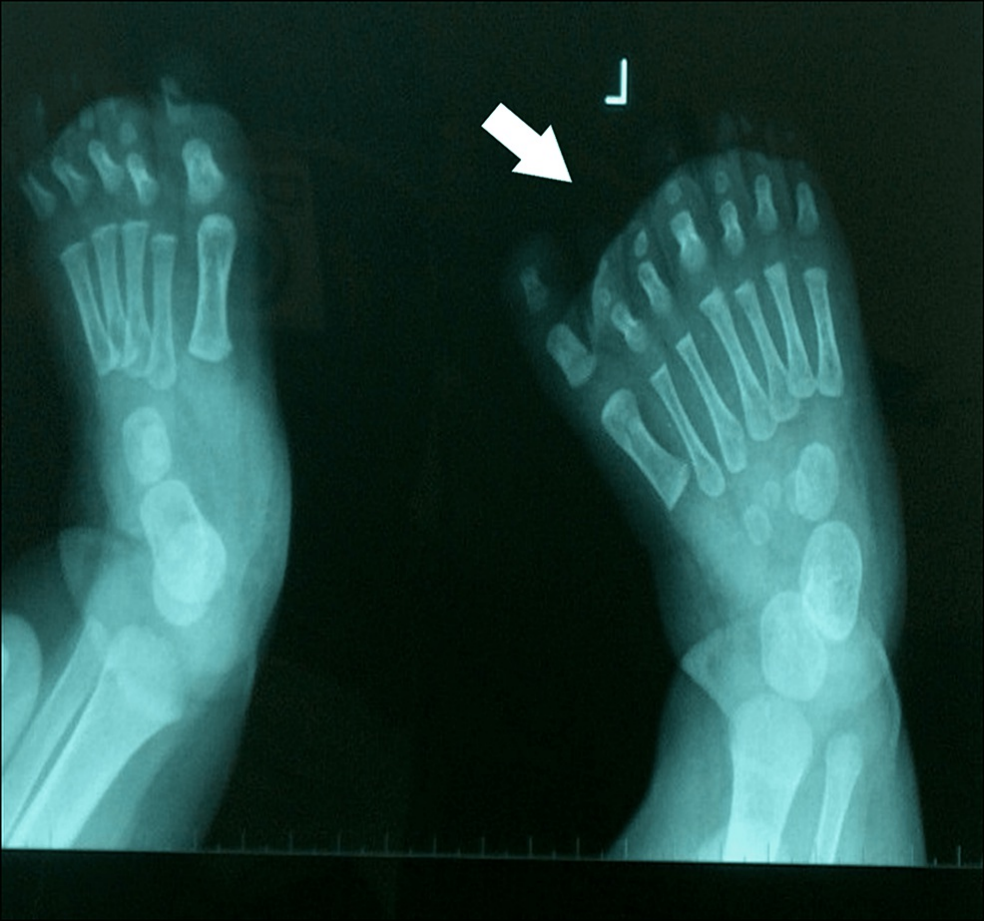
X-ray of the second patient's mirror foot with central polydactyly.
